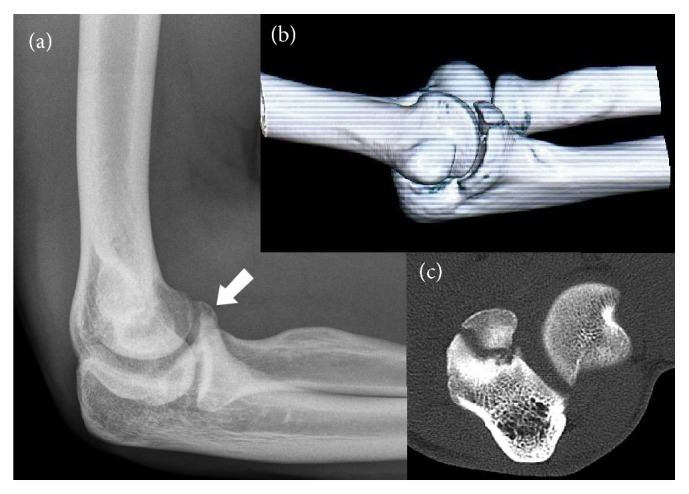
Imaging of the elbow at diagnosis. (a) X-ray: irregularity of the anterior elbow joint. (b) Three-dimensional CT-scan reconstruction of ancient coronoid process fracture. (c) Axial view of elbow on CT-scan.
